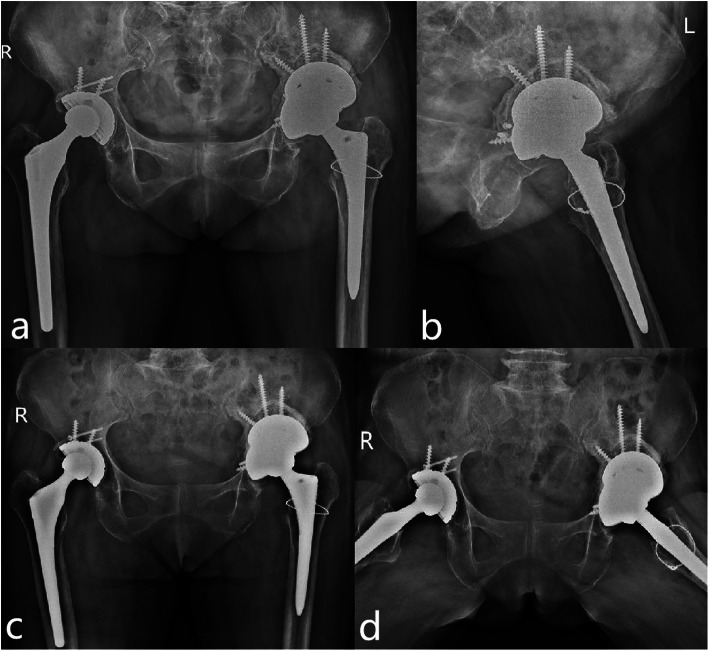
Radiographs (a, b) show double-TM cups buttress bring the hip COR to an anatomic position. Postoperative radiographs (c, d) show no prosthesis loosen or dislocation at the 1 year follow-up after revision surgery
